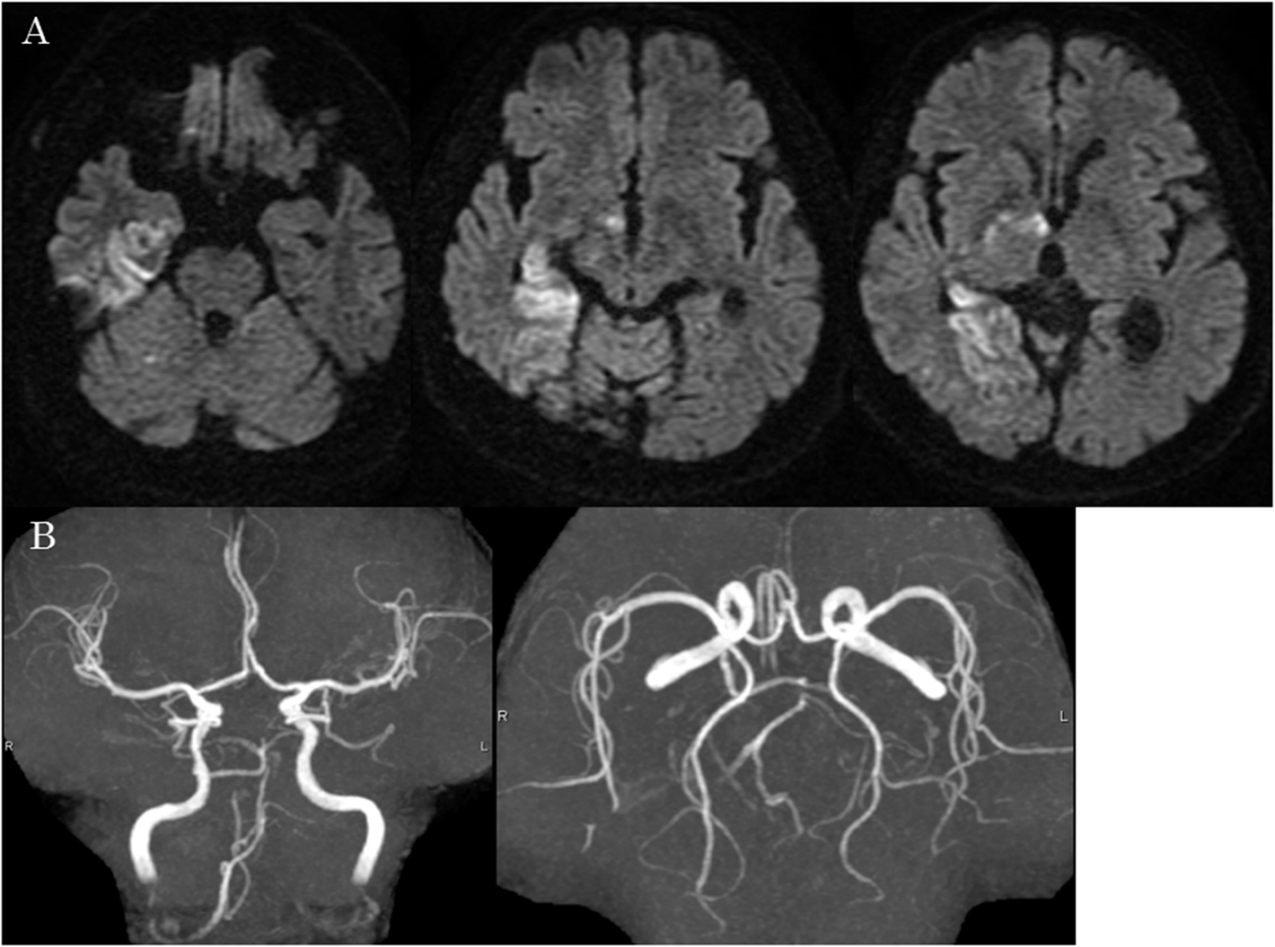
Postoperative MRI/MRA. a There were no new acute ischemic lesions on postoperative MRI DWI. b All cerebral vessels including PPTA were represented on the postoperative MRA
